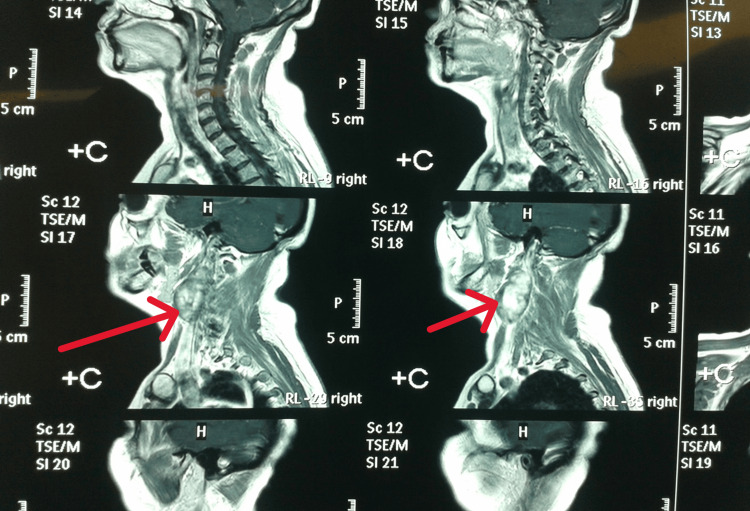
Sagittal MRI section of a cervical schwannoma case
